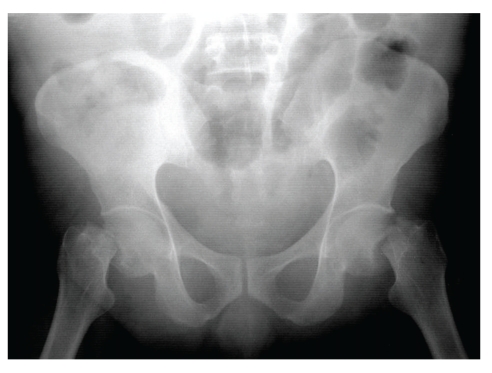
Radiograph of the pelvis showing bilateral fracture of neck of femur.
